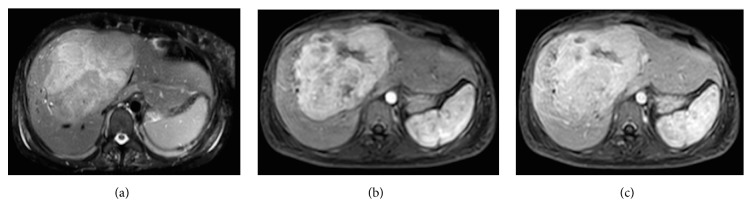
Abdominal magnetic resonance images: (a) T1W, (b) arterial phase, and (c) portal phase. Focal hepatic lesion with lobulated borders and a 13 cms of diameter which has signal heterogeneity in T1 and T2 sequences, with restrictive regions in the diffusion sequence; the lesions are highlighted in the arterial phase; they are practically isointense in the portal and late phase. Irregular hypointense linear area which corresponds to a scar zone.
